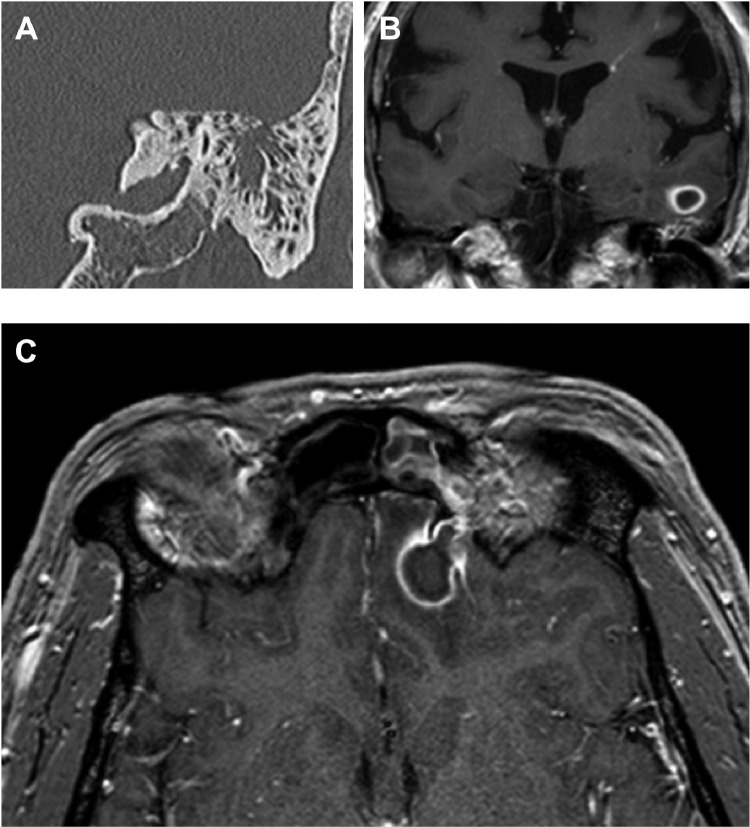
Neuro-imaging to demonstrate the anatomical relationship between sinus infection and brain abscess. A: Coronal high resolution CT image (shows left-sided otomastoiditis with focal breach of the bony roof of the mastoid. B: Coronal, gadolinium enhanced T1-weighted image, performed in the same patient 10 days after the CT scan, shows a small cerebral abscess inferiorly in the left temporal lobe adjacent to the infected mastoid (for panels A and B, patient ID: BA04). C: In a different patient, an axial, gadolinium enhanced T1-weighted image demonstrates a left frontal brain abscess secondary to adjacent left frontal sinusitis with focal breach of the posterior wall of the sinus (for panel C, patient ID: BA21).
